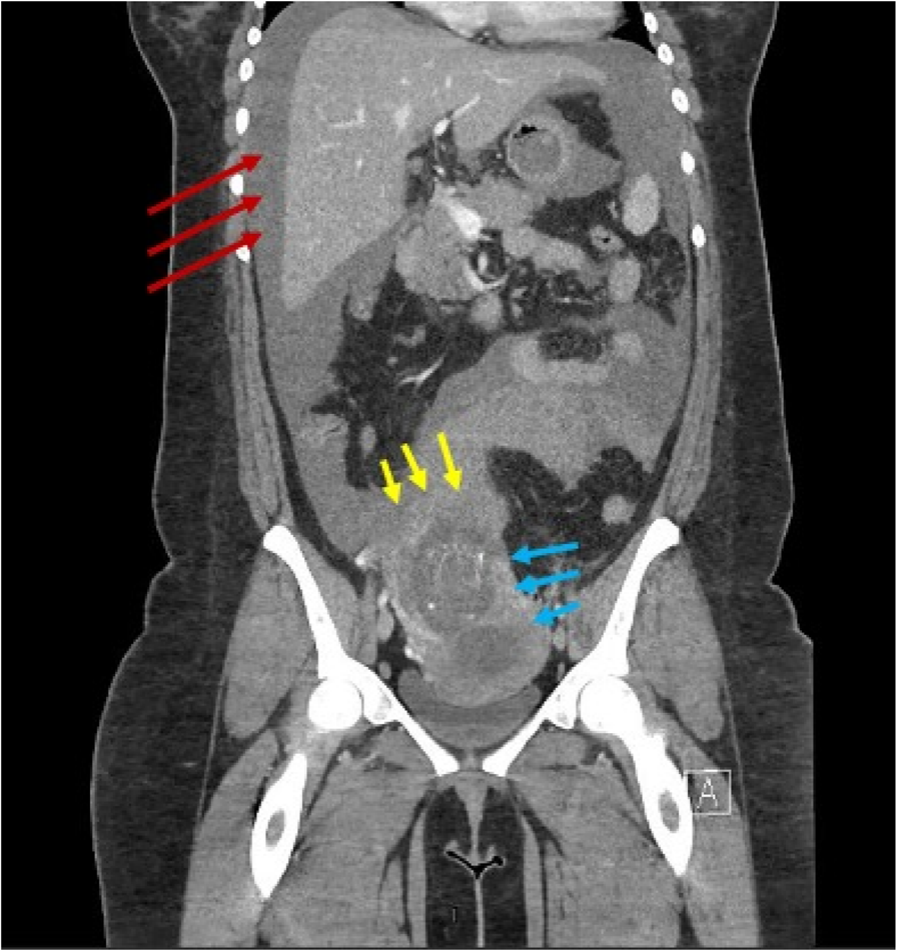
CT scan of the abdomen and pelvis in the coronal plane. The scan revealed large volume hemoperitoneum (red arrows), location of interstitial ectopic pregnancy (blue arrows), an empty uterine cavity (blue arrows), and the area of suspected rupture (yellow arrows)
